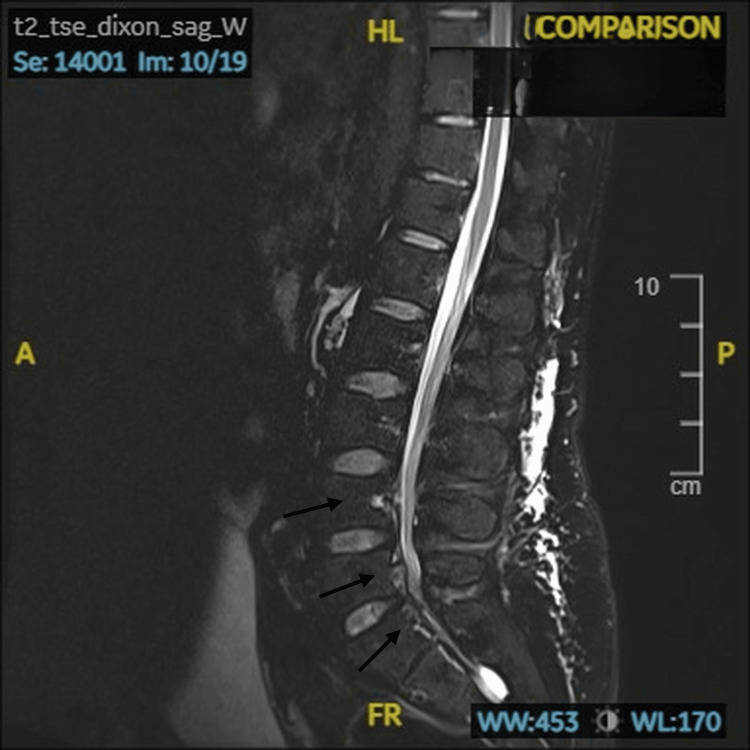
Prominent veins seen in the anterior epidural space at L4-L5 and S1 levels Sagittal view of MRI demonstrating prominent veins seen in the anterior epidural space at L4-L5 and S1 levels with the absence of disc herniation.
